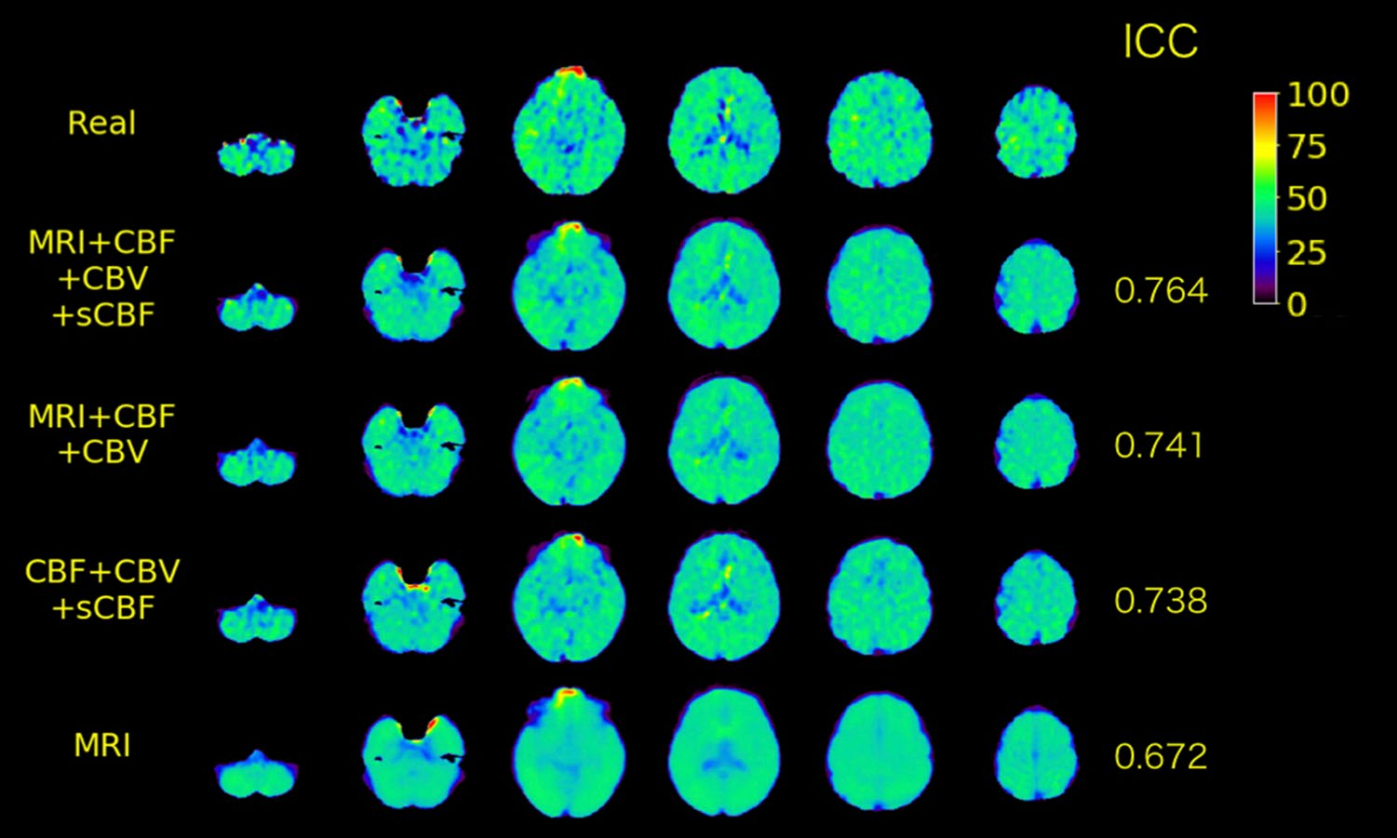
Real and predicted OEF maps for a case (77 years old, male, right internal carotid artery occlusion), with the highest ICC by the full model (MRI + CBF + CBV + sCBF) in the validation data set. The map on the top indicates the real OEF map. The maps in the three rows in the center indicate the OEF maps predicted by the model with the top-three mean ICC among the validation data set (full model; MRI + CBF + CBV; CBF + CBV + sCBF). The bottom map indicates the OEF maps predicted by the model with the worst mean ICC (MRI). ICC values for each model are also shown on the right
